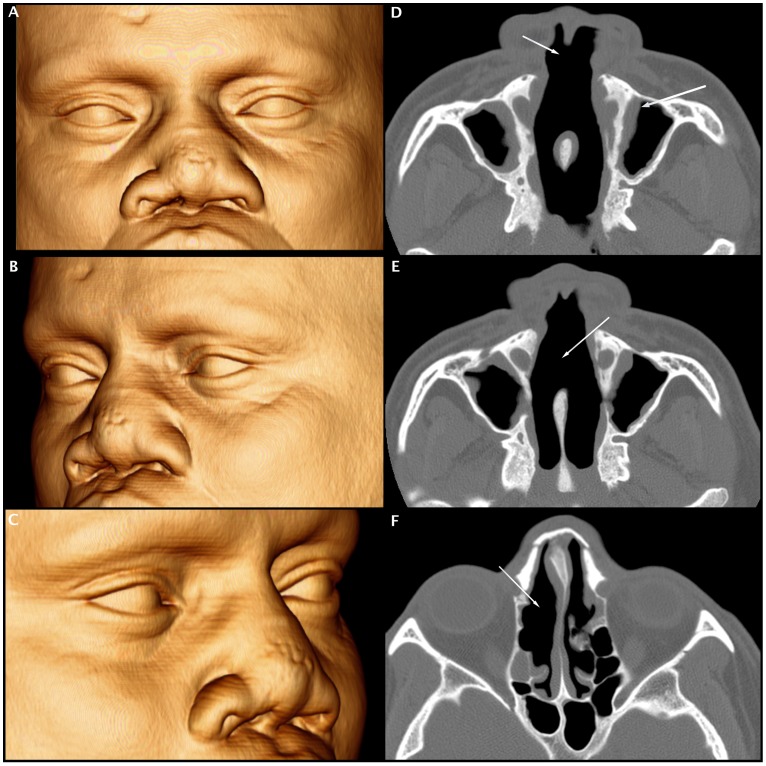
Volume-rendered 3D images of multi-slice CT data (A, B and C) and axial CT scans of the bone window (D, E and F) showing diffuse thickening of the nasal wings with collapse of the nasal pyramid (A, B and C). Axial CT scan shows erosion of the nasal septum (D and E), erosion of the anterior ethmoid cells (F) associated with mucous thickening of the nasal fossae (F), and partial opacification of the maxillary sinuses with thickening of their bony walls (osteitis-D and E).
